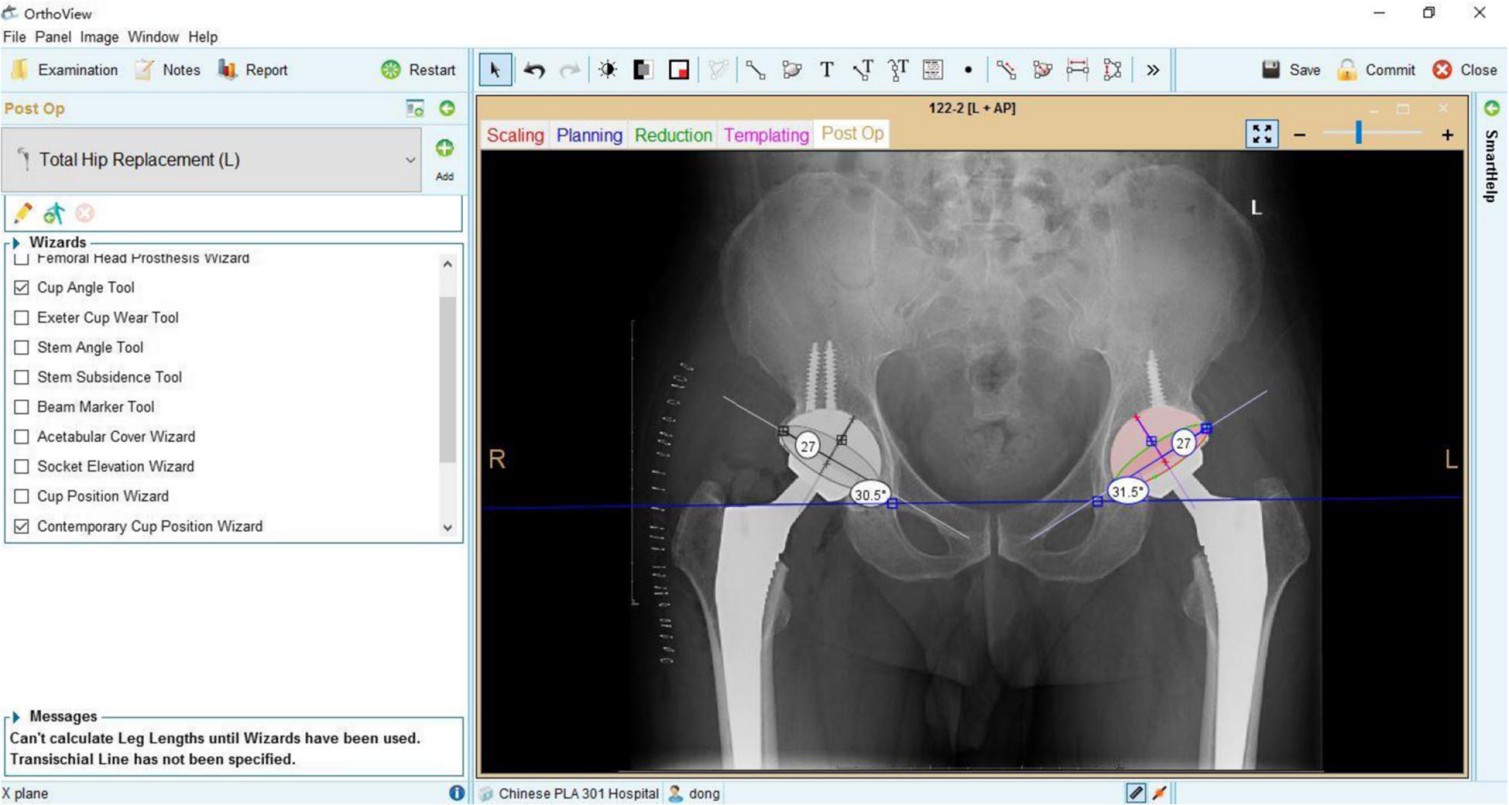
Method for measuring acetabular anteversion and inclination on an AP pelvic radiography using the Orthoview Digital Planning Software system
